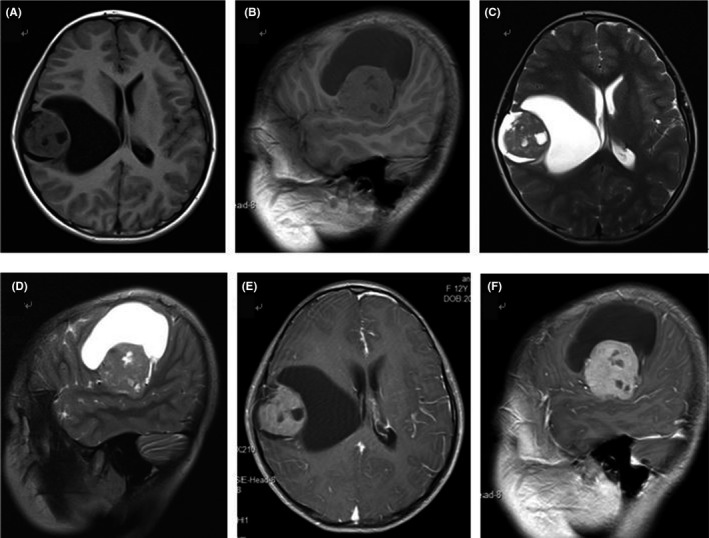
MRI showed a lesion 7.3 × 6.2 × 6.8 cm in size in the temporal lobe with mixed long‐T1 signal (a, b), mixed long‐T2 signal (c, d), and heterogeneous enhancement in the solid part (e, f)
